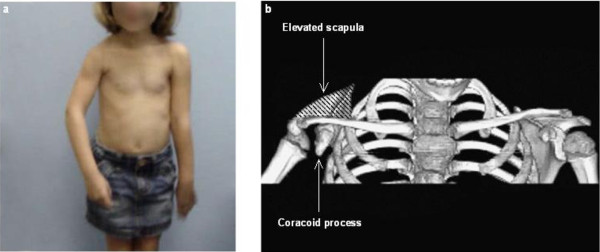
Panel a: A patient with residual brachial plexus injury typically presented with fixed medial rotation contracture of the affected shoulder. Panel b: A 3D CT image from the same patient showing elevated scapula and elongated coracoid process (directed more towards the humeral head) of the affected shoulder.
